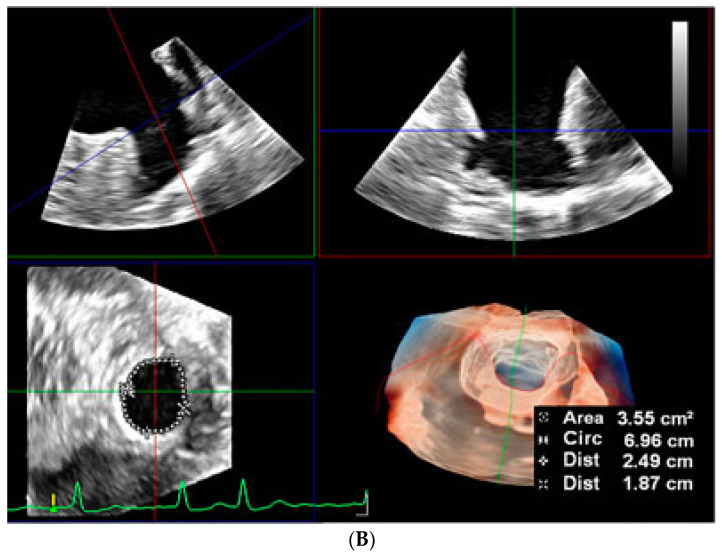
(A) Transoesophageal echo (TOE) image showing the potential device landing zone in the 0°, 45°, 90°, and 135° views. It should be measured from the origin of the circumflex artery to the roof of the LAA at least 10–20 mm below the ligament of Marshall/coumadin ridge. Depth is measured from the plane of landing zone to the LAA apex. Adapted with permission from Ramchand et al. [29]. (B) Three-dimensional LAA measurement. MultiVue mode is employed on the LAA 3D Zoom image, and at the end of the systole, the landing zone positioning line (blue line) in the LAA sagittal plane (upper left) and coronal plane (upper right) are adjusted to obtain the LAA landing zone cross-section (lower left), and the LAA LZ maximum diameter (the first Dist value obtained), minimum diameter (the second Dist value), area, and circumference (Circ) are manually measured. The lower right figure shows 3D downward view of the LAA LZ and the measurement results. Adapted with permission from Sun et al. [27].
